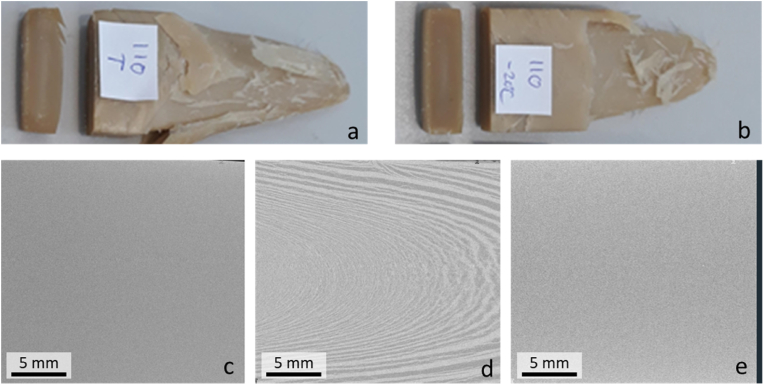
Effect of freezing on wedge. A) picture of fresh sample; B) picture of defrosted sample; C) X-Ray reconstruction of a fresh sample; D) X-Ray reconstruction of a frozen sample; E) X-Ray reconstruction of a defrosted sample.
